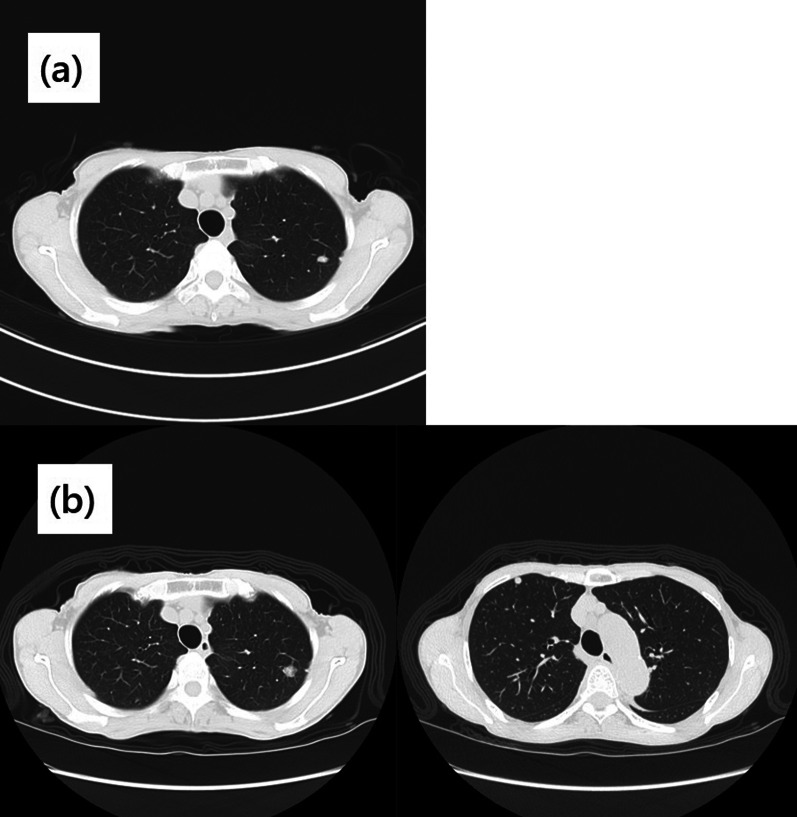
Computed tomography showing a pulmonary nodule. a 22 months after surgery; b 25 months after surgery
